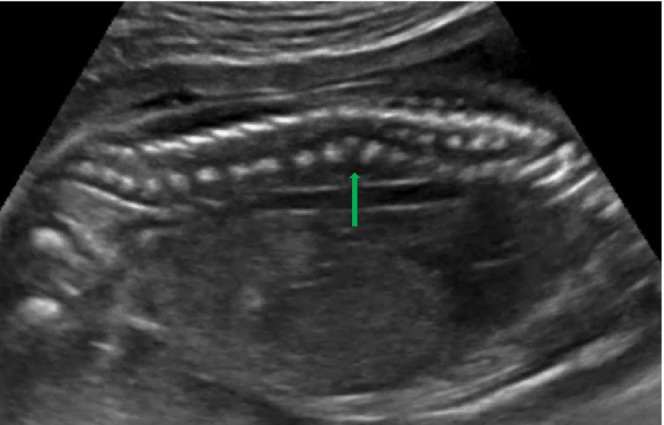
Ultrasound image showing angulation in fetal spine in sagittal plane (green arrow).
